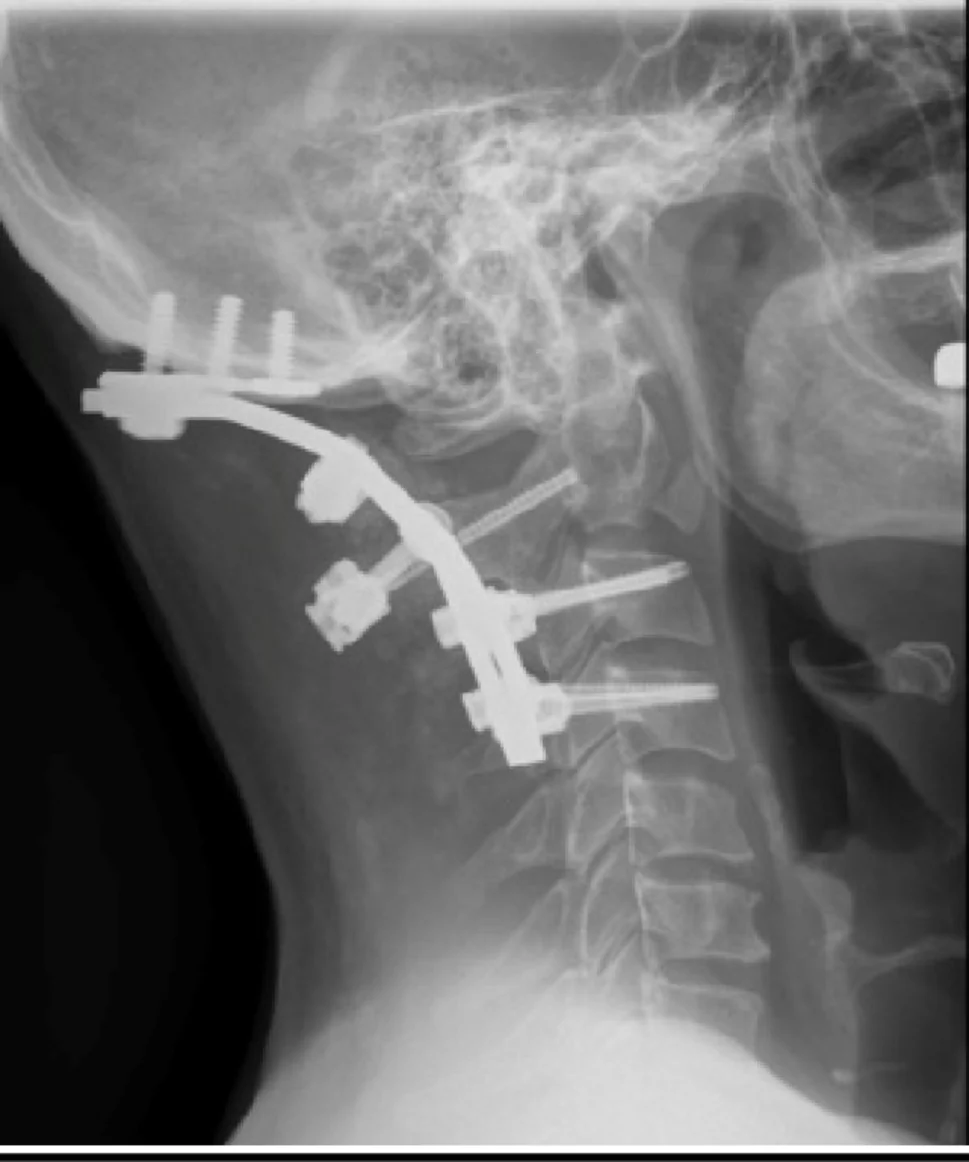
Lateral X-ray of occipitocervical fusion (C0-C2-rt.-C3-C4) for C1 ring AO type B N0 fracture (= Gehweiler III), C2 AO type B N0 and Dens type III fracture (same patient as in figures 4–9). *C*,* cervical spine*
